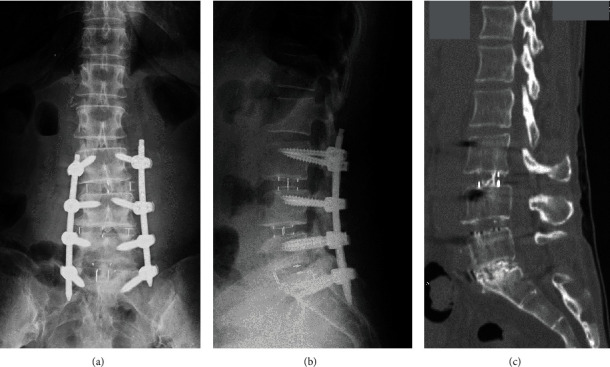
Postoperative radiographs and computed tomography (CT) image of the lumbar spine of a 49-year-old woman with degenerative lumbar disease. (a) anteroposterior view X-ray, (b) lateral view X-ray, and (c) sagittal CT image two years following surgery.
